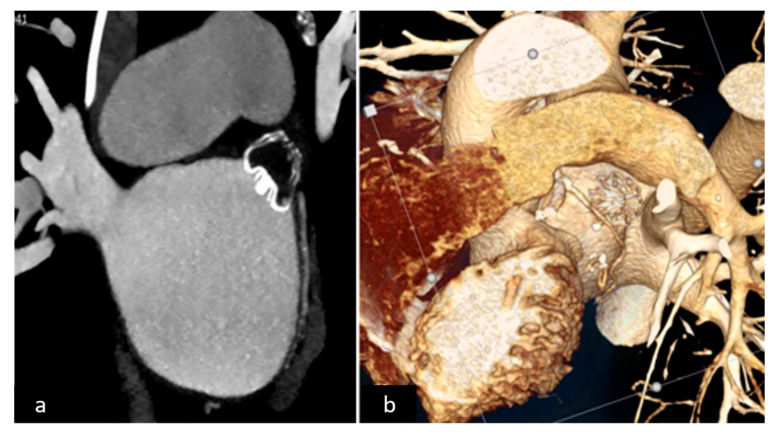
(a) 2D CT scan endocardial device, (b) 3D CT scan reconstruction.
